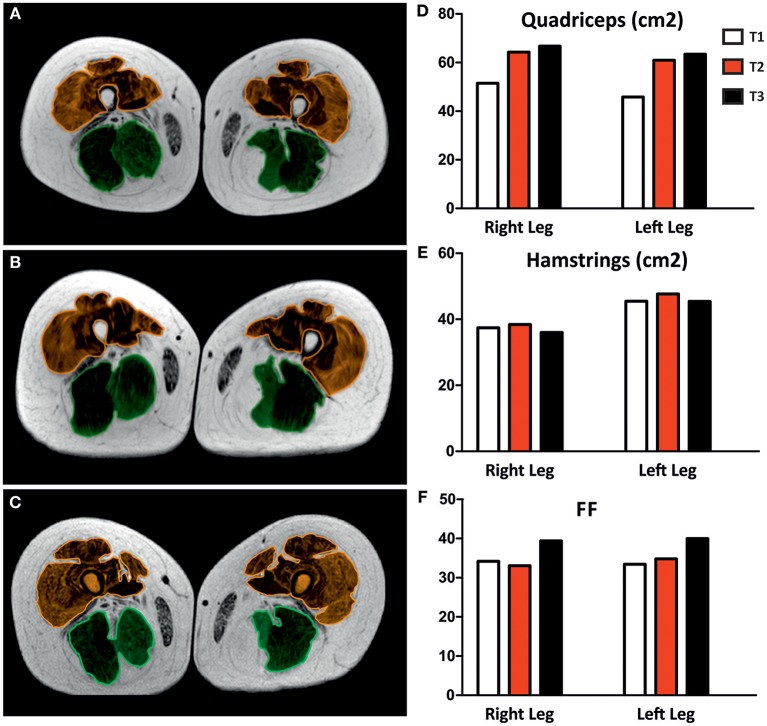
Magnetic Resonance Images (MTI) of thigh muscles. MRI was performed after 5 (A; T1), 14 (B; T2), and 26 months (C; T3) of home-based FES. The cross-sectional area of quadriceps (brown) and hamstrings (green) muscles was estimated and plotted in (D,E), respectively. Histogram in (F) show the variation of fat fraction (FF) during FES treatment.
